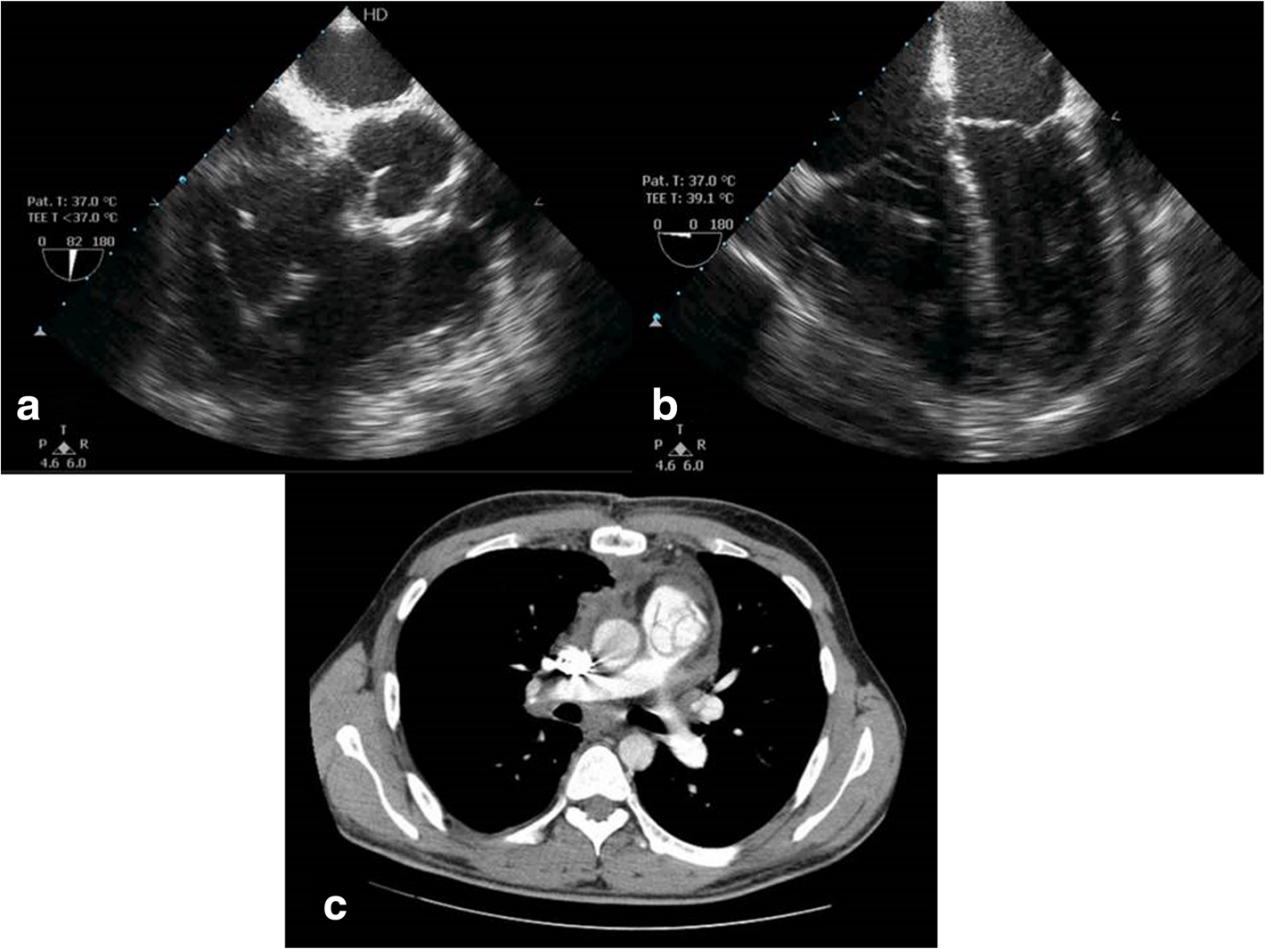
a and b Postoperative transesophageal echocardiography demonstrates no cardiac mass, the biologic prosthesis in pulmonary position, free tricuspid valve, and dilation of the right ventricle. c Postoperative chest computed tomography demonstrates good contrast visualization of the trunk and the two branches of the pulmonary artery
